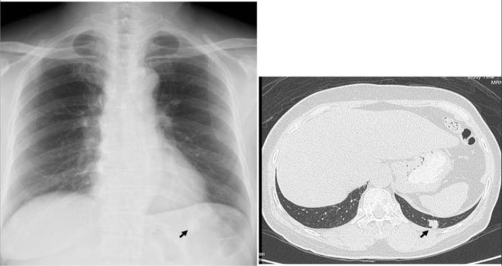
Chest X-ray and CT in Case 1 revealed a 1 cm nodular shadow over the supra-diaphragmatic area of the left lung.
